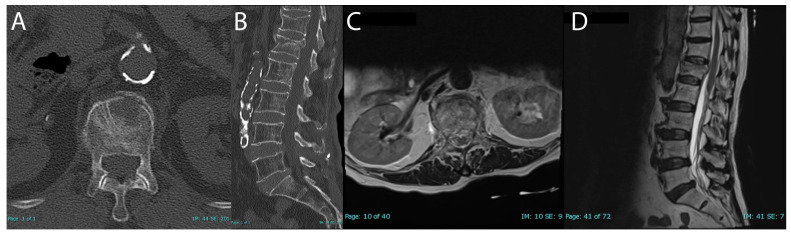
Preoperative CT and MRI assessment of burst fracture (A–D); admission CT axial (A); CT sagittal (B); admission MRI axial T2 (C); MRI sagittal T2 (D).
